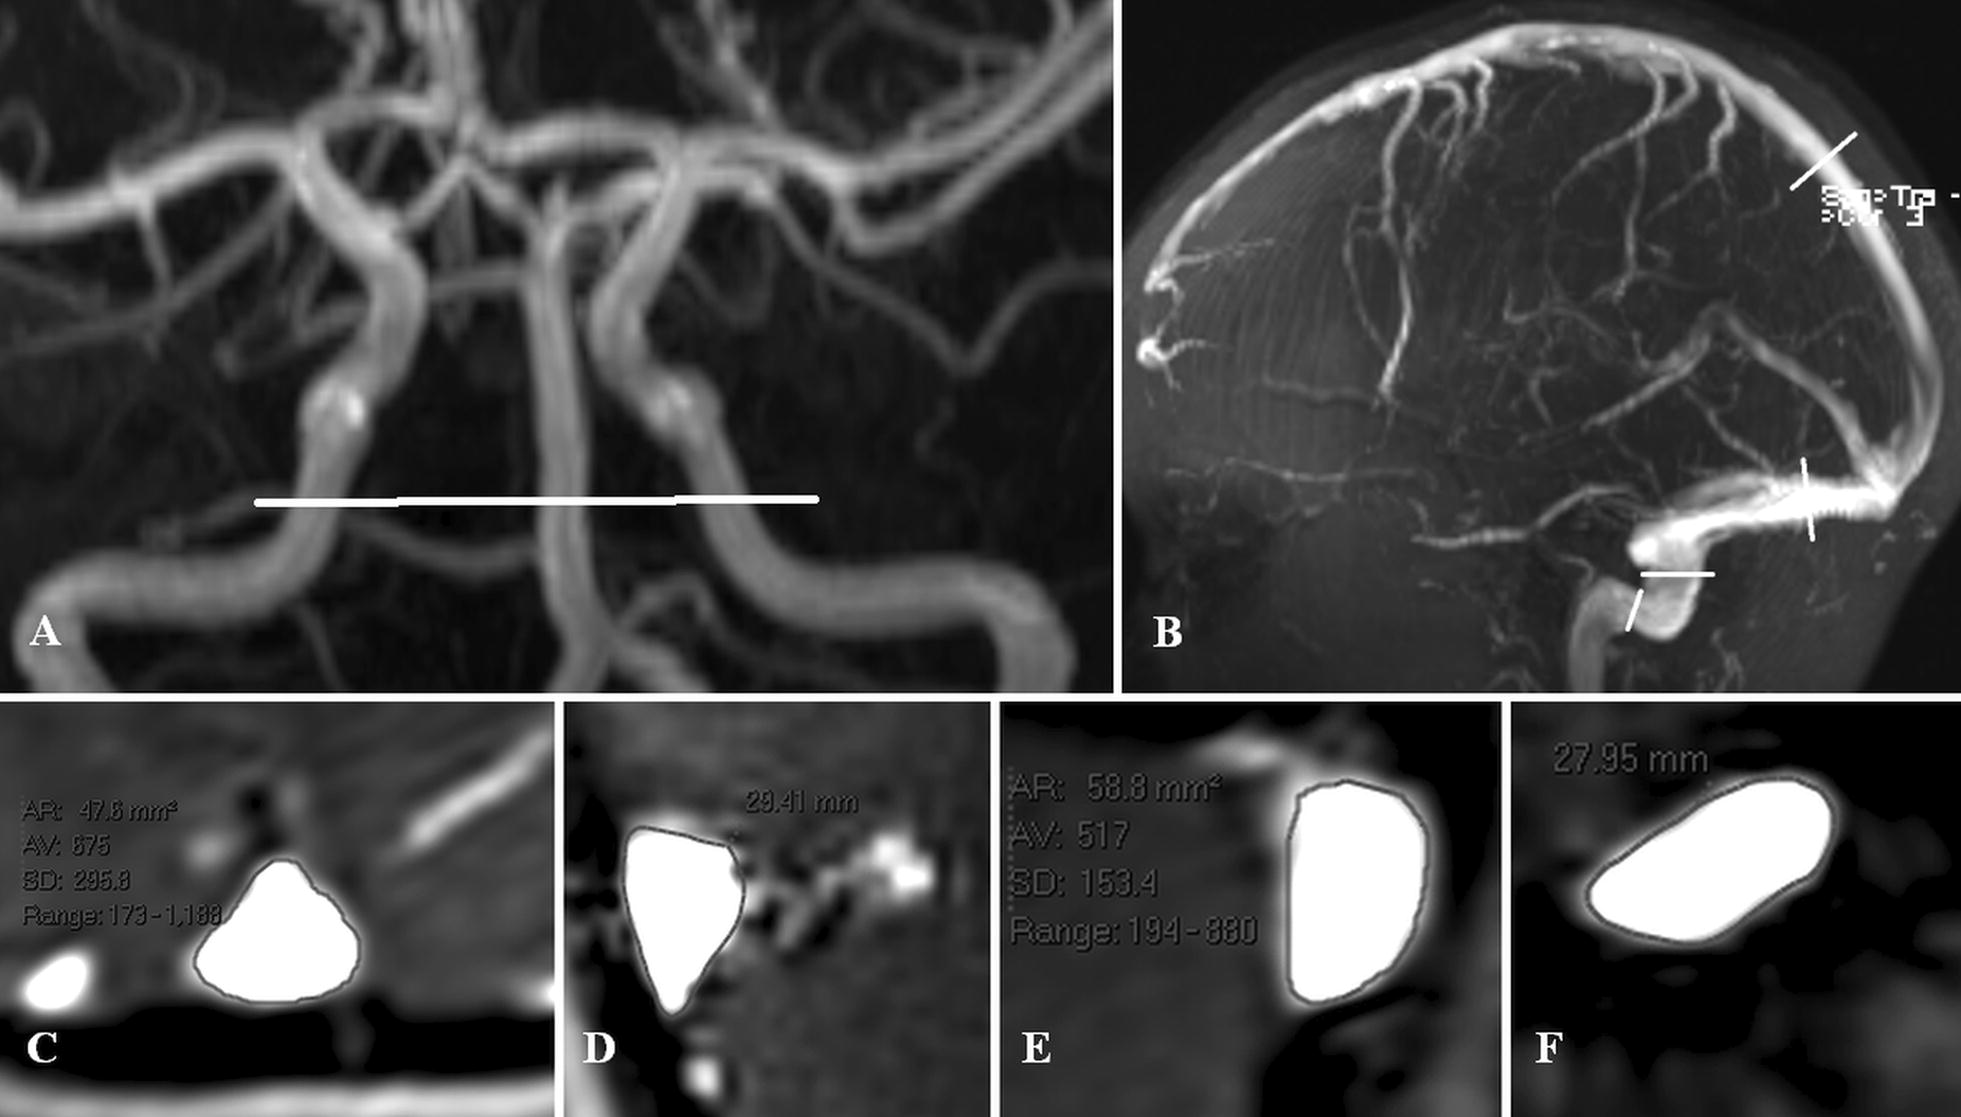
Site of flow acquisition and venous sinus measurement in a control patient. a An MRA of a 4.7 year old male with seizures who had a normal MRI. The while line indicates the level of the flow quantification acquisition. The acquisition crosses both carotid and basilar arteries at the skull base. b An MRV of the same child with while lines showing the sites of the cross-sectional measurements. From superior to inferior these are the sagittal sinus, transverse sinuses, vertical portion of the sigmoid sinuses and the horizontal portion of the sigmoid sinuses. c The cross-sectional image of the sagittal sinus which is triangular. d The cross-sectional image of the right transverse sinus which is triangular. e The cross-sectional image of the left vertical sigmoid sinus which is oval. f The cross-sectional image of the horizontal portion of the sigmoid sinus which is oval
